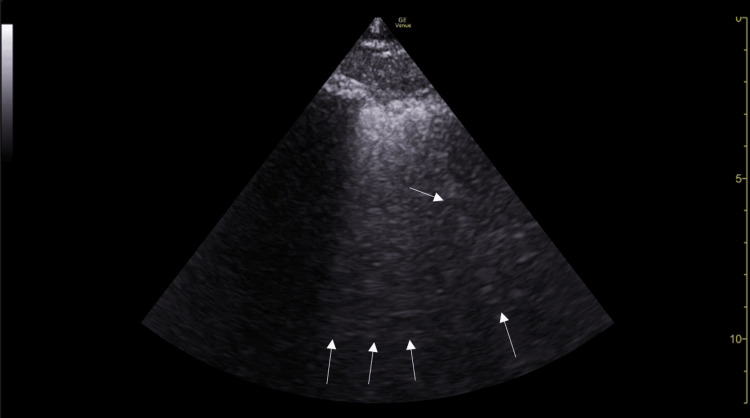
Example lung ultrasound image with multiple B-lines present (white arrows), representative of likely pulmonary edema.
